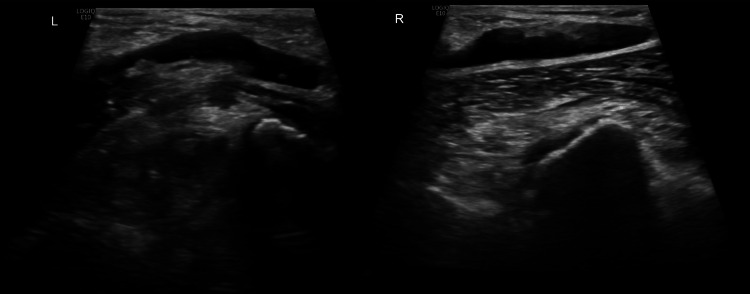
Echo-Doppler ultrasound of the lower limbs Heterogeneous luminal filling and lack of compressibility on the left (L) and right (R) popliteal veins, aspects compatible with bilateral deep vein thrombosis
